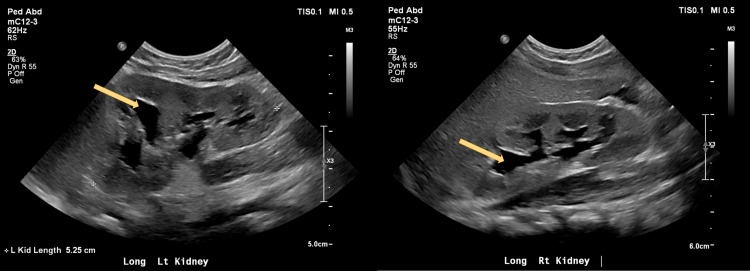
Renal ultrasound showing hydronephrosis in bilateral kidneys
